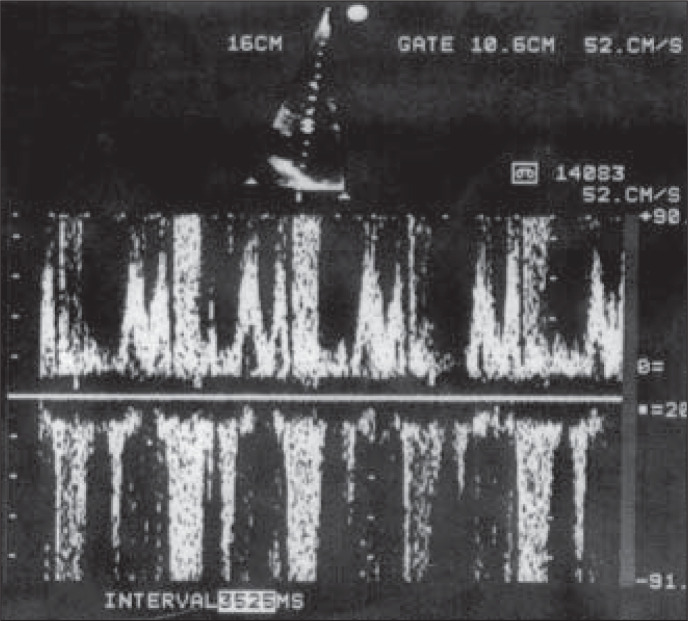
Echocardiogram showing mitral regurgitation.
